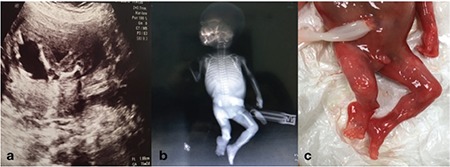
The right leg. a) Sonography of the right leg, showing the shortening of the right femur and abnormally angulated tibia, b) X-ray of the lower limb shows proximal focal femoral deficiency, short and angulated tibia and absence of fibula, c) post-abortus material visualization confirming the abnormalities of the right leg and right foot
